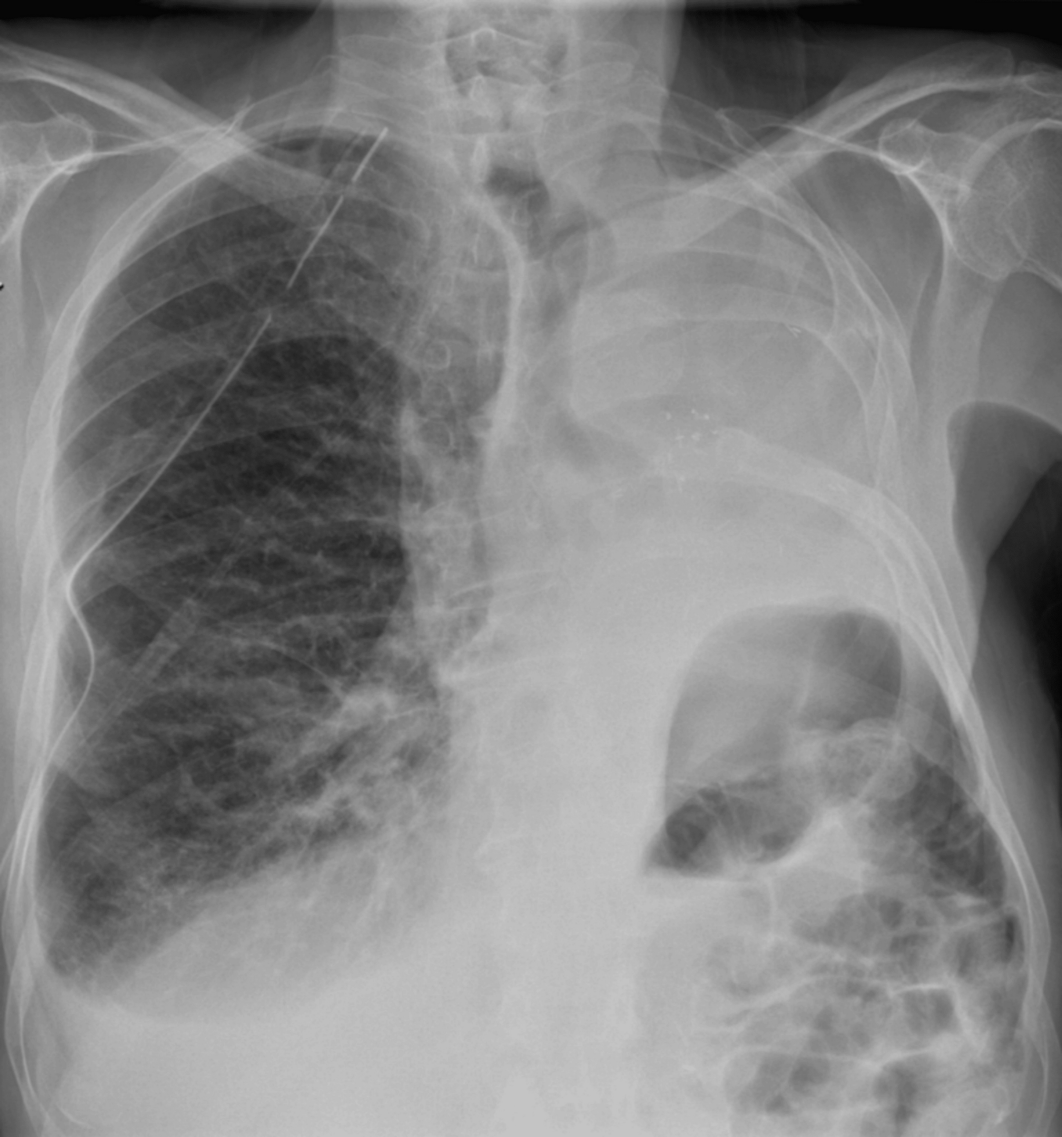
Postoperative chest radiograph. Postoperative chest radiograph demonstrating full expansion of the right lung following surgery, with no evidence of residual pneumothorax and a small residual pleural effusion.
